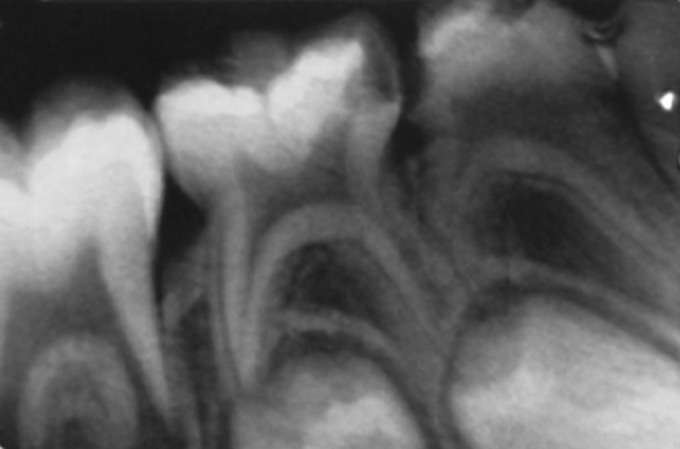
All patients were radiographed for accurate diagnosis
